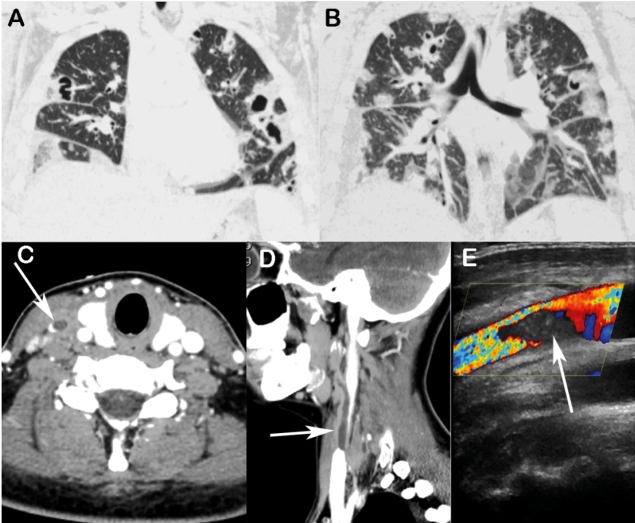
Chest computed tomography (CT) with coronal reconstruction (A and B) demonstrating multiple bilateral pulmonary nodules, most of which were cavitated and associated with areas of consolidation. Right pleural effusion should also be noted. Contrast-enhanced CT of the neck region with axial (C) and sagittal (D) reconstruction showing a hypoechoic thrombus in the right internal jugular vein (arrows). Color Doppler imaging (E) also demonstrating the thrombus (arrow).
